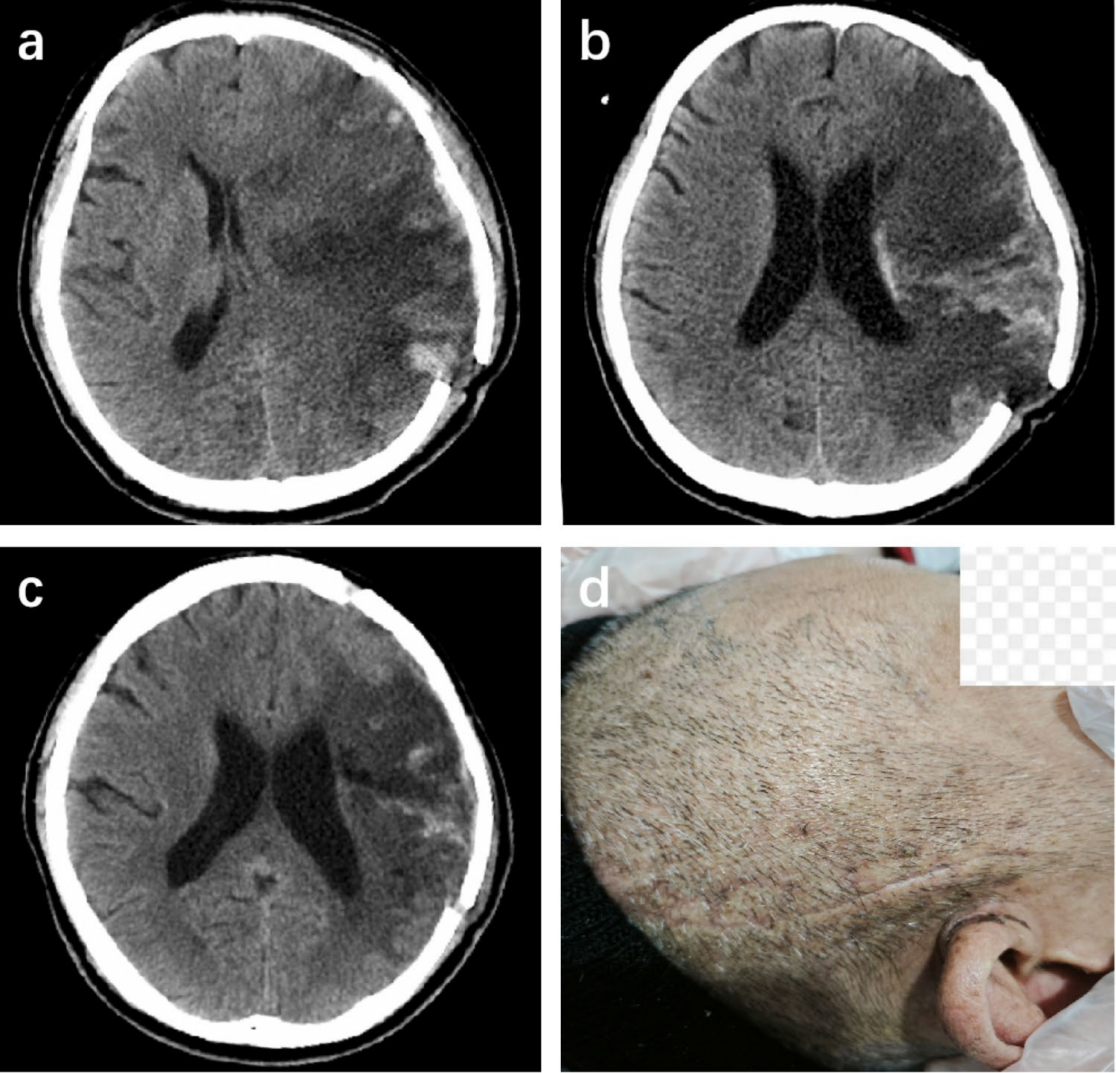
Postoperative imaging and clinical follow-up. a Head CT on postoperative day 3, demonstrating significant cerebral edema with good elevation of the floating bone flap. b Follow-up CT on postoperative day 9, showing resolution of cerebral edema while the bone flap remains elevated. c Follow-up head CT at 3 months, showing satisfactory anatomical reduction of the bone flap with no subsidence. d Clinical photograph at 3 weeks, showing an excellent cosmetic outcome with no signs of bone flap subsidence
